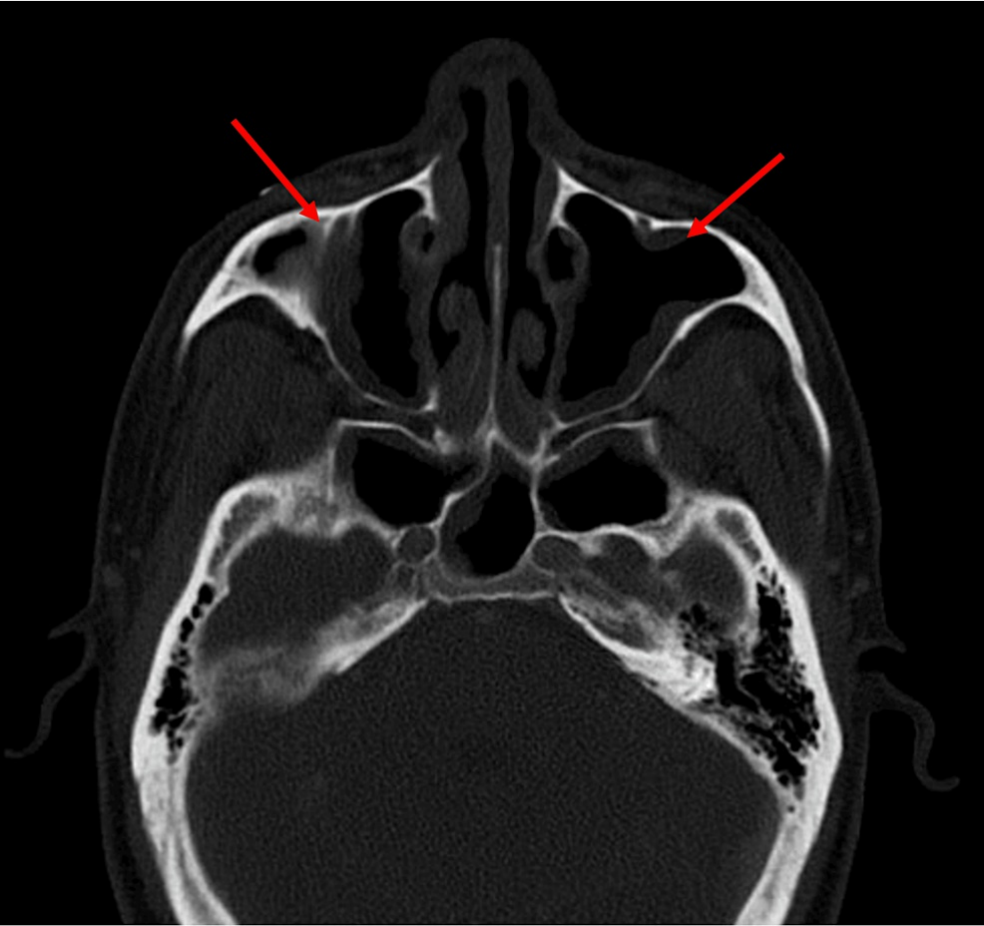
CT sinuses with IV contrast. The radiographic image shows moderate mucosal thickening of bilateral maxillary sinuses with partial obstruction of left > right maxillary ostia (red arrows). CT: computed tomography
